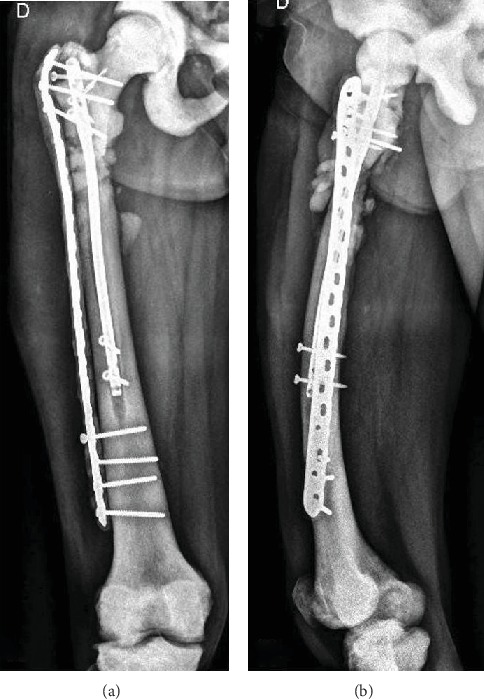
Postoperative anteroposterior (a) and lateral (b) radiographs of the first revision surgery described.
